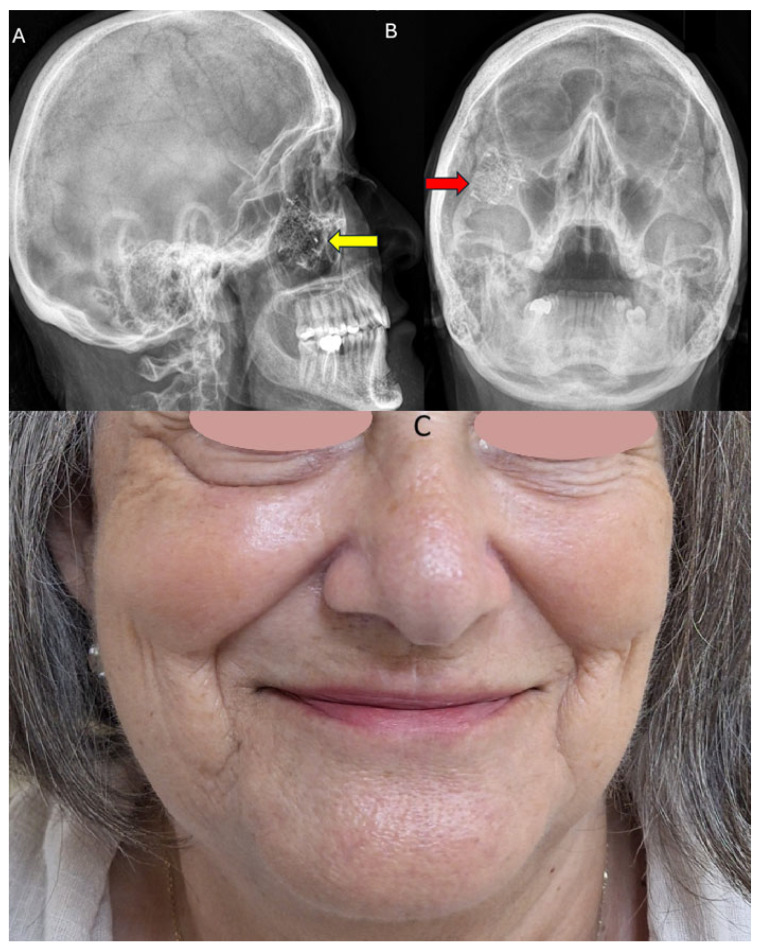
Control post-operative lateral ceph (yellow arrow) radiograph revealed proper mesh alignment. The maxillary sinus is radiolucent and without signs of infection, indicating that it remained intact during the operation (A). Waters’ radiograph revealed a very good bone symmetry and zygomatico-malar prominence position. The titanium mesh can be noticed in the proper position in the body of the right zygomatic bone, transfixed in the infraorbital rim with titanium screws (Modus 2.0, Medartis, Basel, Switzerland) (red arrow) (B). The final result after surgery is excellent. The patient was examined 5 months after surgery in the outpatient clinic. The cosmetic result is outstanding, and the scar is barely visible. The function of the infraorbital nerve was maintained, and midface anatomy was restored to normal without any secondary deficits. The scar is well hidden in the facial wrinkles in relaxed skin tension lines (RSTLs) (C). Facial nerve function is maintained, and facial expression remains the same. The patient remains under clinical observation. A good CT examination, followed by PSI-guided 3D-printed models, grants a safe surgery with good outcomes.
